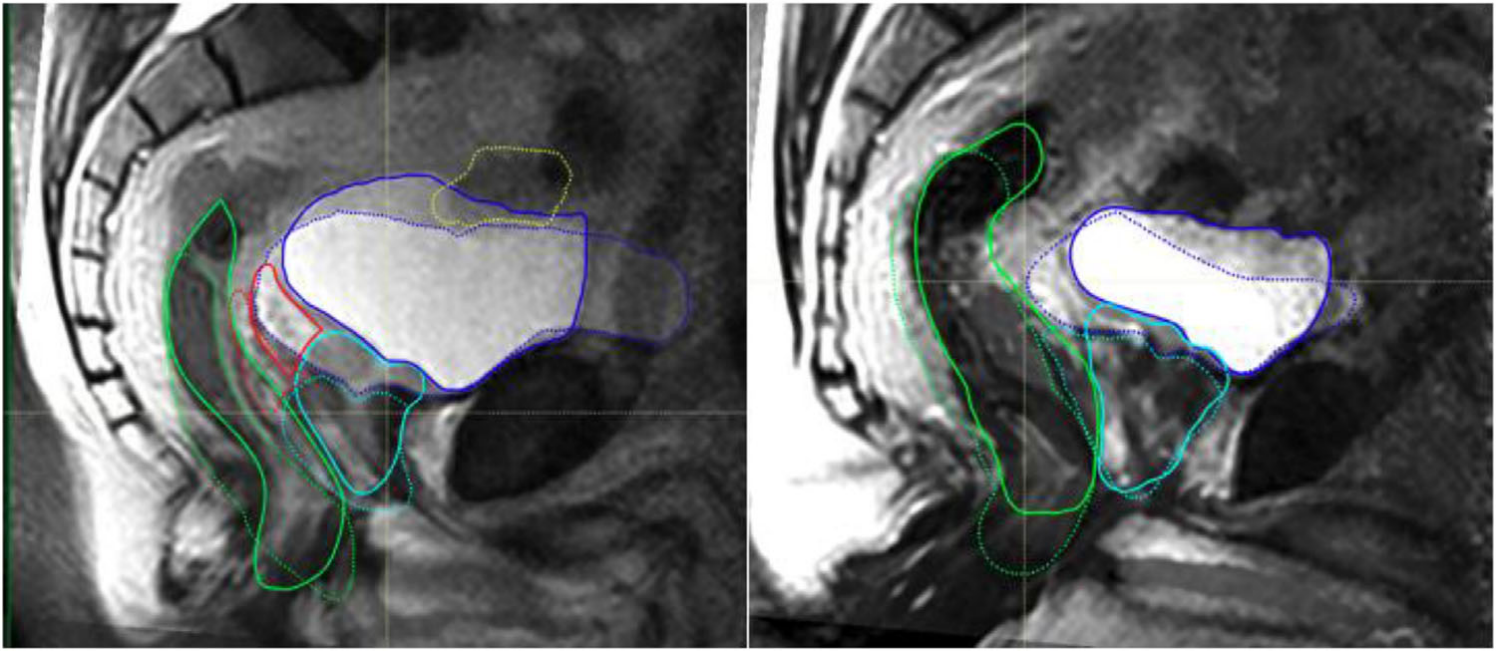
The midline sagittal MRI images for the supine (solid lines) upright (dotted lines) positions for two volunteers. The following organs are shown in both panels—rectum (green), prostate (light blue), bladder (blue) and small bowel (yellow—left panel only).
